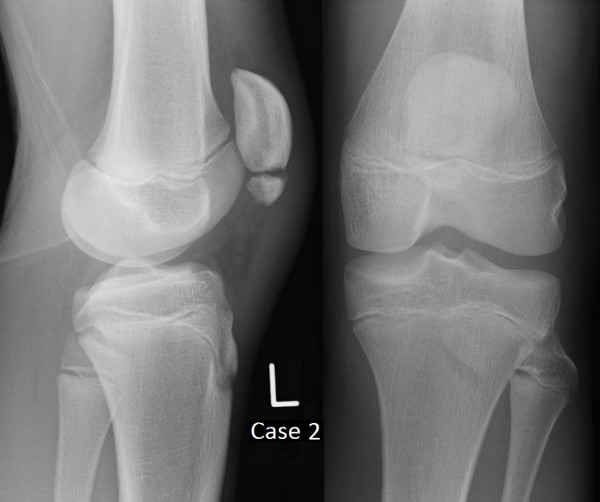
Plain film radiographs of Case 2 at presentation.
